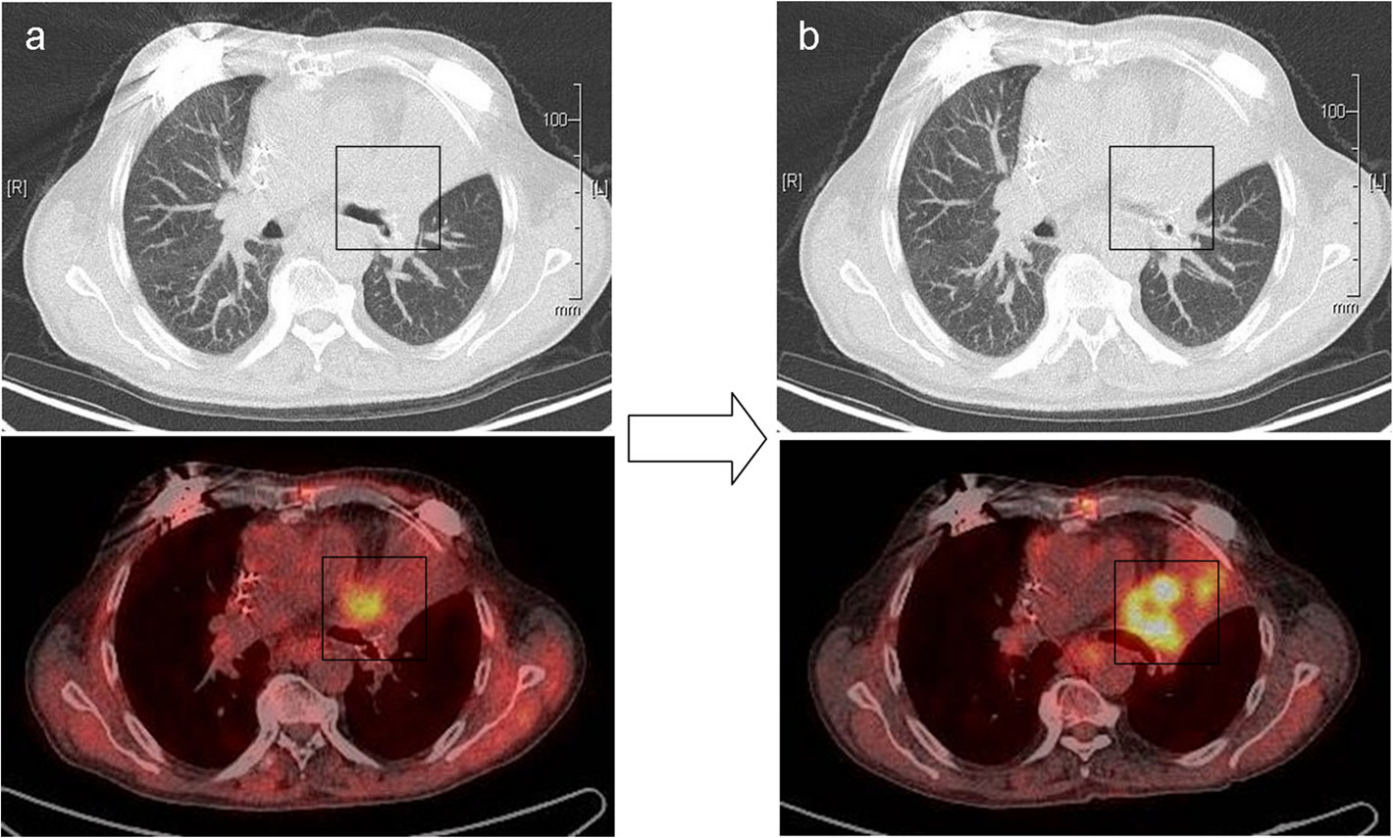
Radiological follow-up staging after radiotherapy: a) significant reduction of tumor size and FDG-uptake in three months after radiotherapy; b) progressive disease in six months with the intrabronchial tumor propagation and increased metabolic activity.
